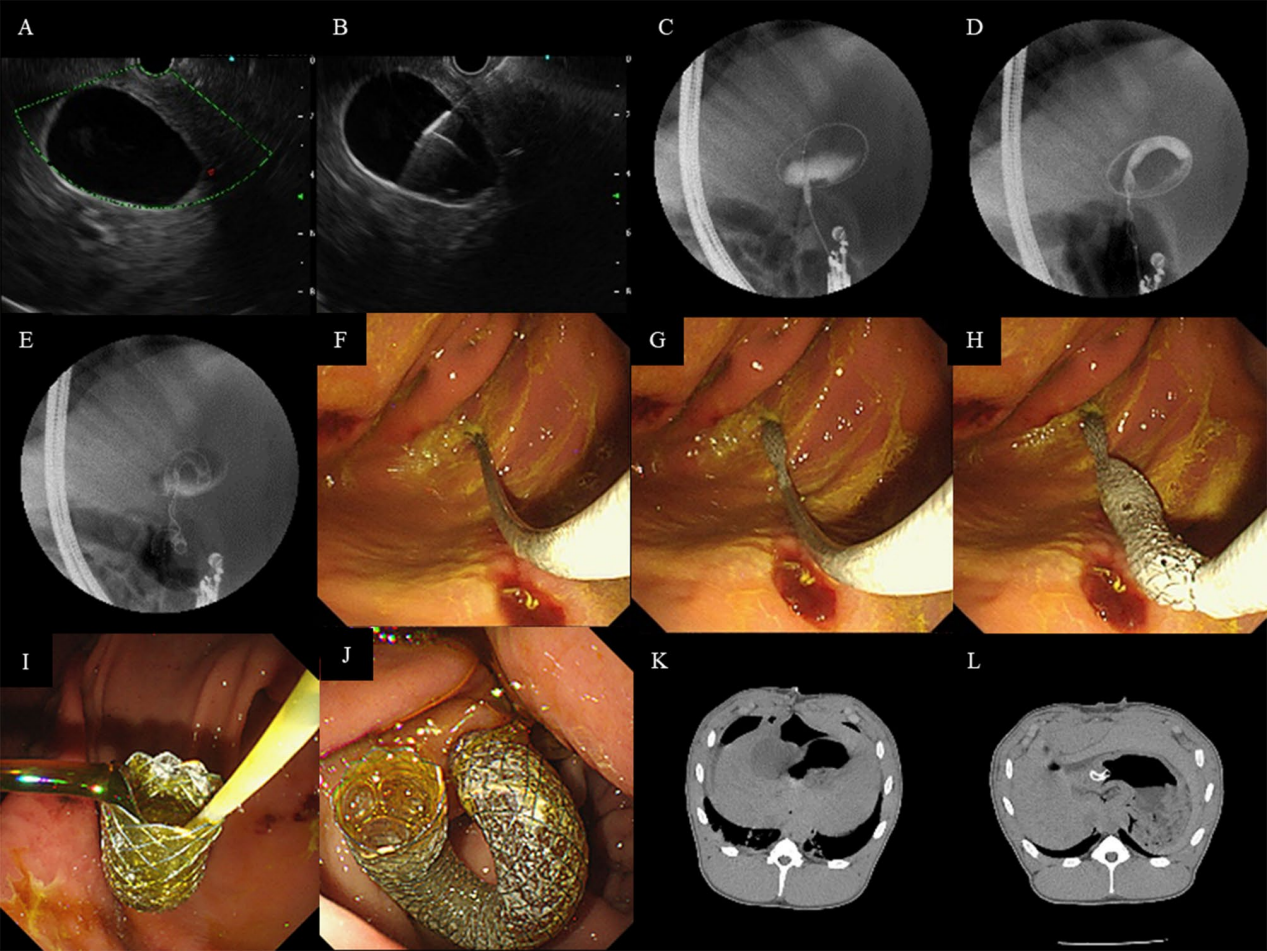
EUS-guided cholecysto-gastrostomy using a novel stent. (A) Assessment of regional vascularity with color Doppler, (B) puncture of GB by a 19G needle under EUS guidance. (C) Balloon dilatation of puncture site. (D–J) Stent deployment under fluoroscopic, EUS and endoscopic guidance. (J) Endoscopic view of the fully deployed stent. (K) Preoperative CT. (L) CT performed at post-operative 2 weeks showing the stent located between GB and stomach.
